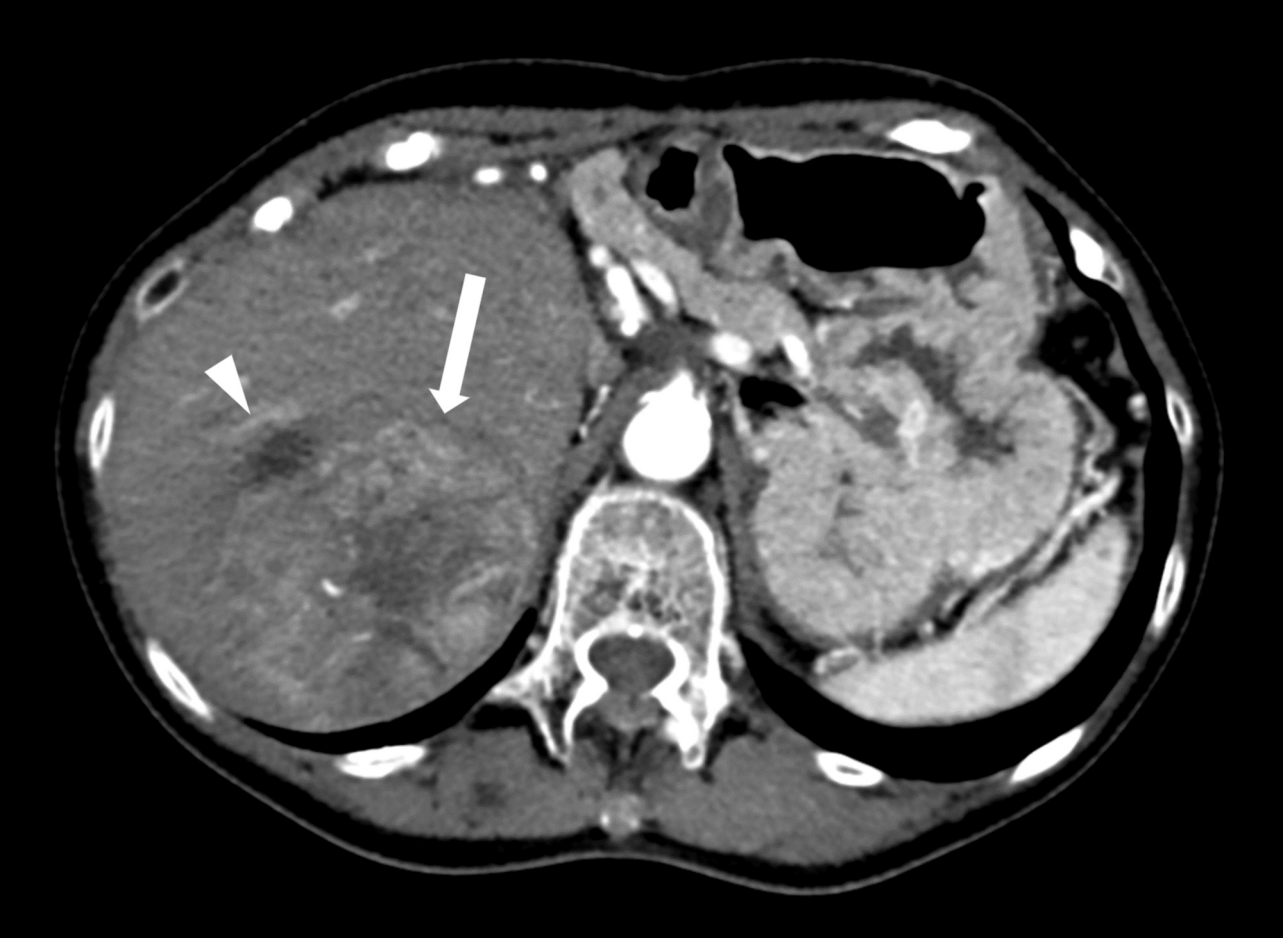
Axial CTA Large, heterogeneously enhancing mass with central necrosis measuring 7.3 x 6.0 cm in the greatest axial dimension (arrow). The lesion is subcapsular and subdiaphragmatic, without extracapsular extension. Partially visualized hypodensity anterior to the lesion is a simple cyst (arrowhead). CTA: computed tomographic angiogram
